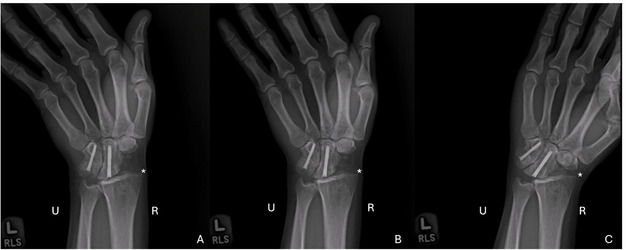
Left, supine on hand table in wrist traction tower. Eight‐week postoperative digital dynamic radiographs showing resected radial styloid (*) and impingement‐free range of motion in (A) ulnar deviation, (B) neutral, and (C) radial deviation. (R, radial; U, ulnar.)
